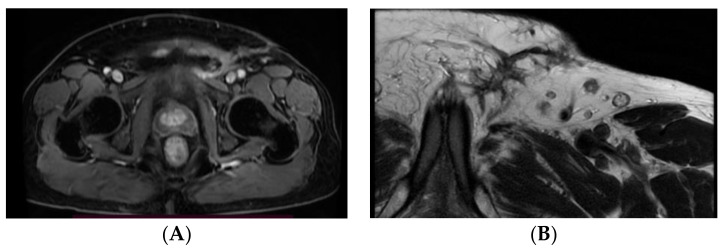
Postoperative MRI aspect of the left inguinal area—axial sections at different levels showing inflammatory modifications (A) and several lymph nodes (B).
